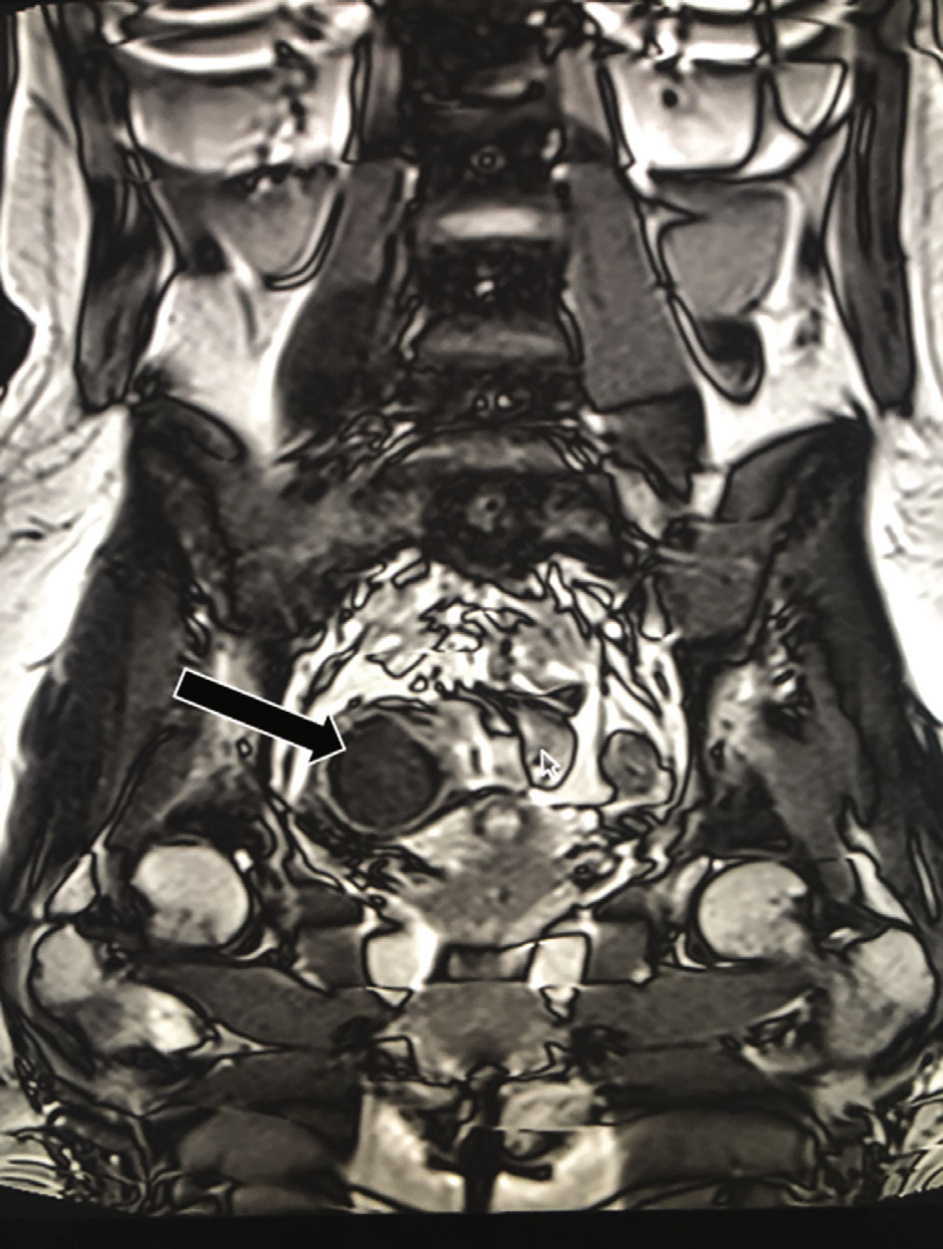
Magnetic resonance imaging showing a cyst at the right iliac fossa.
